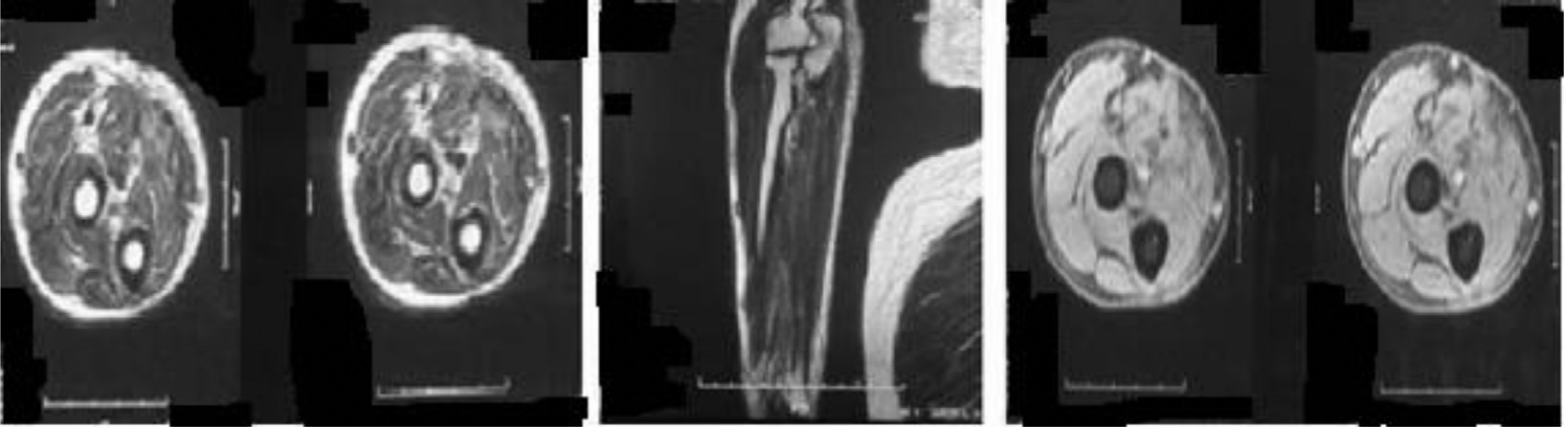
Follow-up MRI sequences showing no signs indicative of tumor recurrence.
